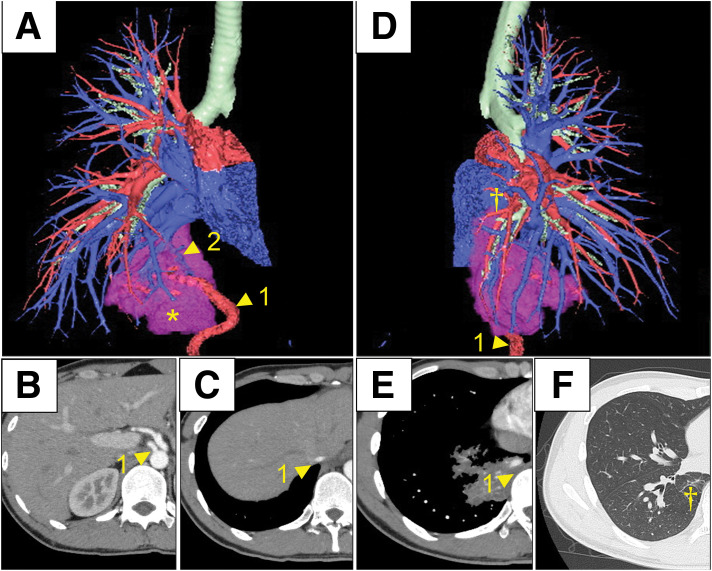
3D-CT findings. (A) 3D-CT (anterolateral view): arrowhead 1, an aberrant systemic artery arising from the abdominal aorta and supplying the lesion; arrowhead 2, venous drainage into the basal pulmonary vein; *, the sequestered area. (B, C) Axial contrast-enhanced CT demonstrating a tubular enhancing vessel (1) coursing from the abdominal aorta toward the lesion. (D) 3D-CT (posterior view): †, no identifiable segmental bronchus entering the lesion, consistent with sequestration. (E) Axial lung-window CT showing an intralesional aberrant systemic artery (1). (F) Axial lung-window CT confirming †, absence of a bronchus corresponding to B10 and lack of communication with the tracheobronchial tree. *, sequestered area; †, absence of a segmental bronchus entering the lesion
